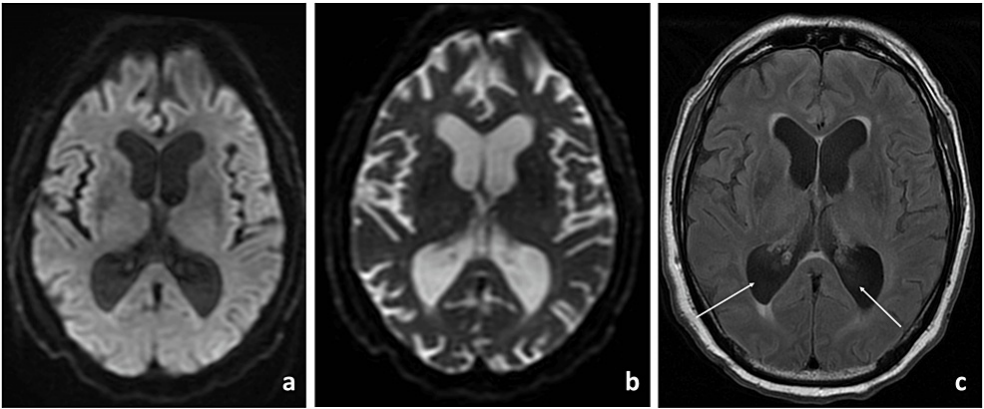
MRI of the brain MRI axial images showed no evidence of infarction on the diffusion-weighted image (a) and apparent diffusion coefficient (b) and showed enlarged ventricles with arrows on the fluid-attenuated inversion recovery image (c). MRI: magnetic resonance imaging
